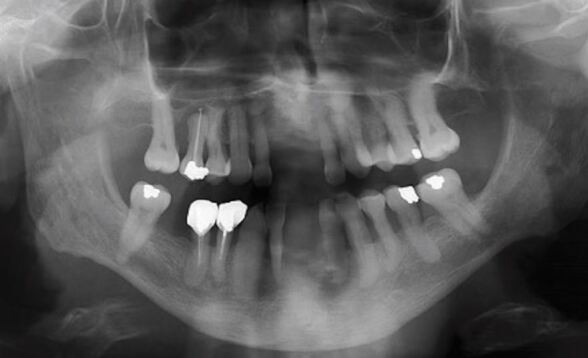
Dental panoramic showing the following radiographic details: endodontic treatment on 15, 44, and 45; presence of periapical radiolucency of the 15 associated with endodontic treatment without associated symptomatology; and bone loss associated with root surface exposure
